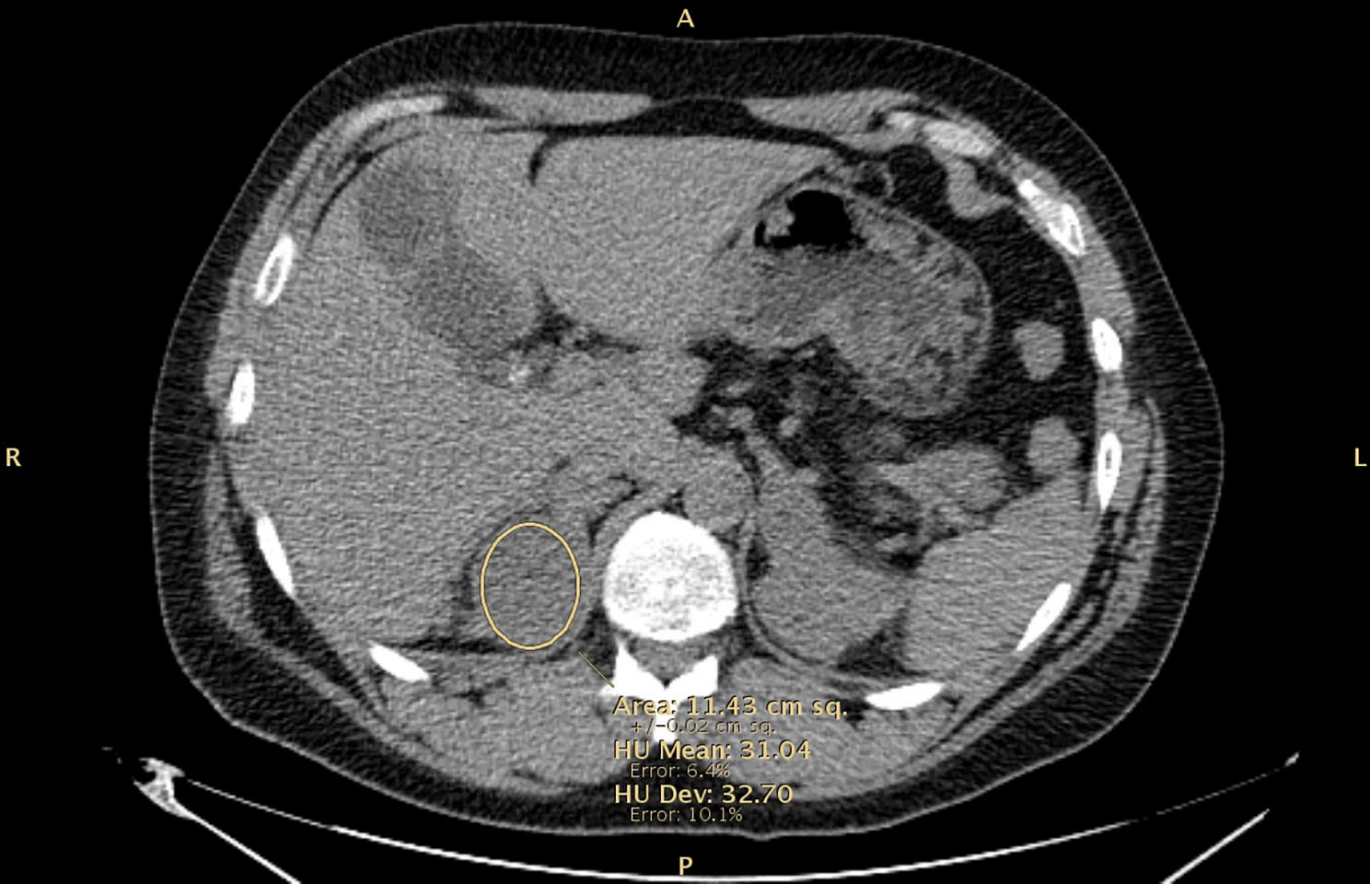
Bilateral adrenal masses at unenhanced CT scan with Hounsfield Unit (HU) of the right adrenal lesion
